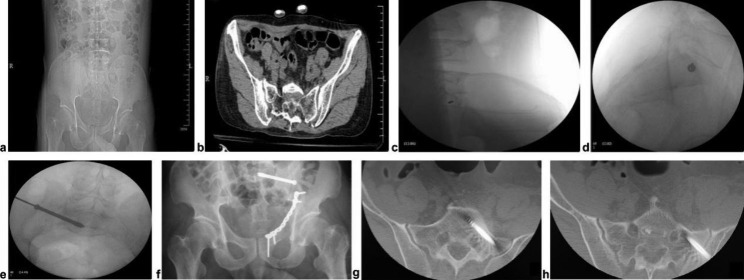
A 43-year-old male with a sacroiliac joint fracture (type III) caused by a fall. Preoperative anteroposterior pelvic radiographs (a) and CT (b) indicate an ipsilateral pubic fracture in a type III sacroiliac joint fracture. Intraoperative X-ray fluoroscopic radiographs (C-arm) during guide wire placement (c), cannulated screw placement (d), and screw insertion (e). Postoperative anteroposterior X-ray (f) and CT radiographs of the pelvis (g, h)
